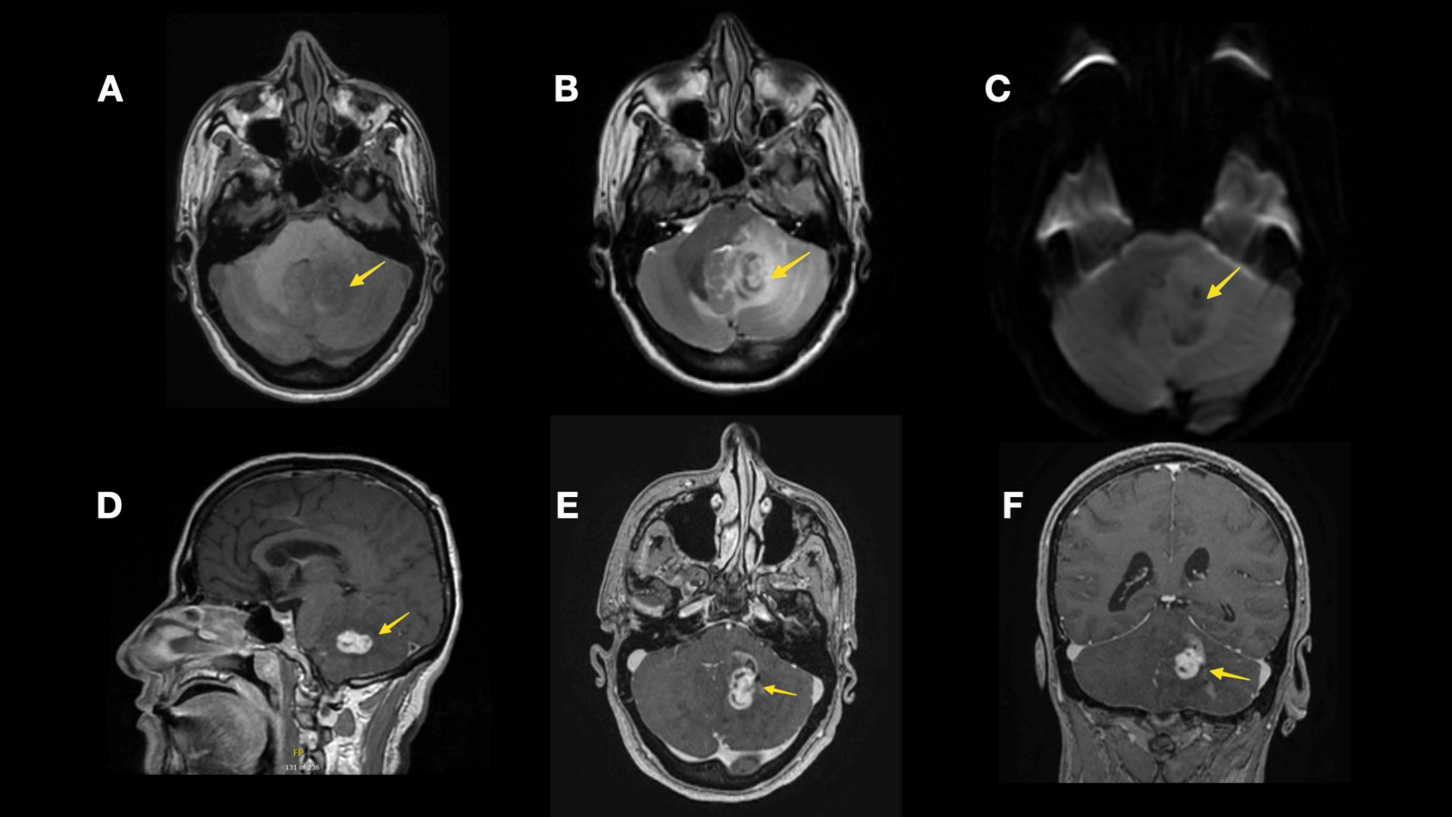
Brain MRI scan images Brain MRI scan revealed an isodense deep left cerebellar hemisphere measuring 2.3 x 1.4 x 1.4 cm lesion (yellow arrow) in the axial T1-WI (A), with marked peri-lesional edema in the axial T2-WI (B). The lesion has no fluid restriction on DWI (C). The lesion demonstrated a homogenous enhancement following intravenous contrast administration in the sagittal (D), axial (E), and coronal (F) views.
